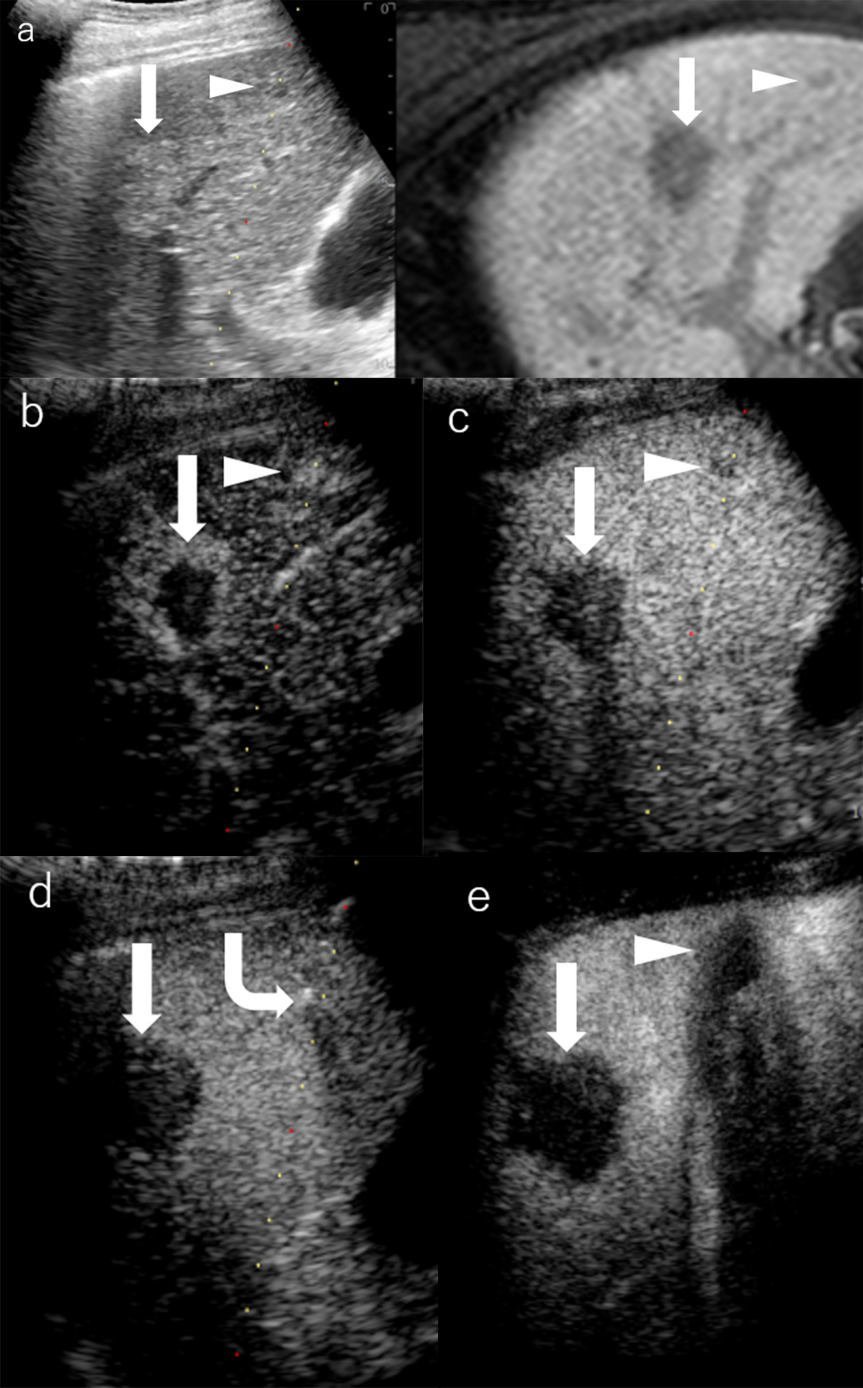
Images of a man in his seventies with a pathological diagnosis of moderately differentiated hepatocellular carcinoma (HCC) and had a history of cirrhosis and HCC. The hepatobiliary phase of EOBMRI (right side), as the reference, was combined with conventional grayscale US (left side), displayed an 8-mm indistinctive hypointense area (the triangular arrow) in segment V on the same screen for the fusion imaging (A). The extrasmall lesion was hypervascular in the arterial phase of Sonazoid-enhanced ultrasound (US) (B), while the post-vascular phase indicated it to be a slightly hypoechoic area (C). Pathway guidance was ready for radiofrequency ablation (RFA) needle manipulation on real-time US (B–D), along with tracking for the metallic needle tip (the curved arrow) (D). The contrast-enhanced US (CEUS) evaluated the target ablation area to be non-enhanced after RFA (E). Arrows indicate the margin of the bigger HCC lesion, which was previously treated by RFA. And Arrowheads indicate the margin of the extrasmall HCC lesion.
